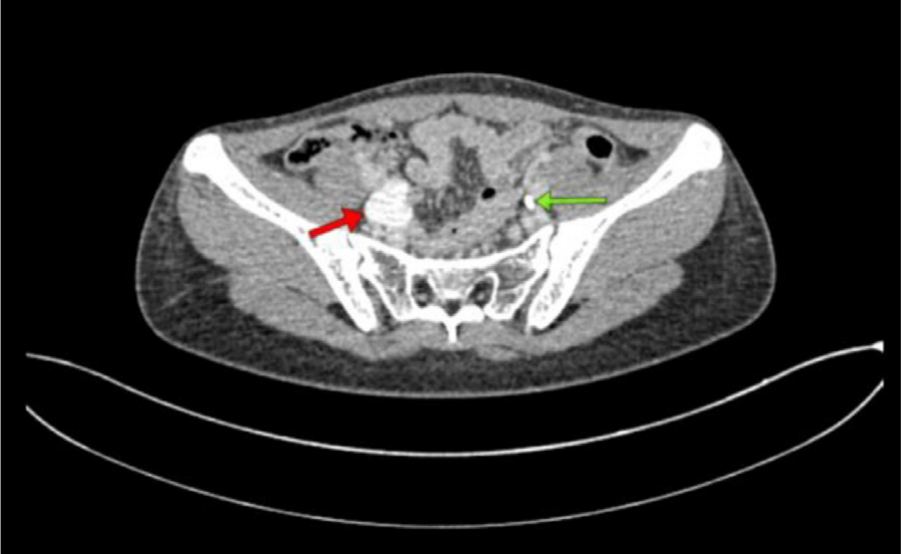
Contrast-enhanced abdominal CT scan: Dilated right ureter (hydroureter) with tortuous.
